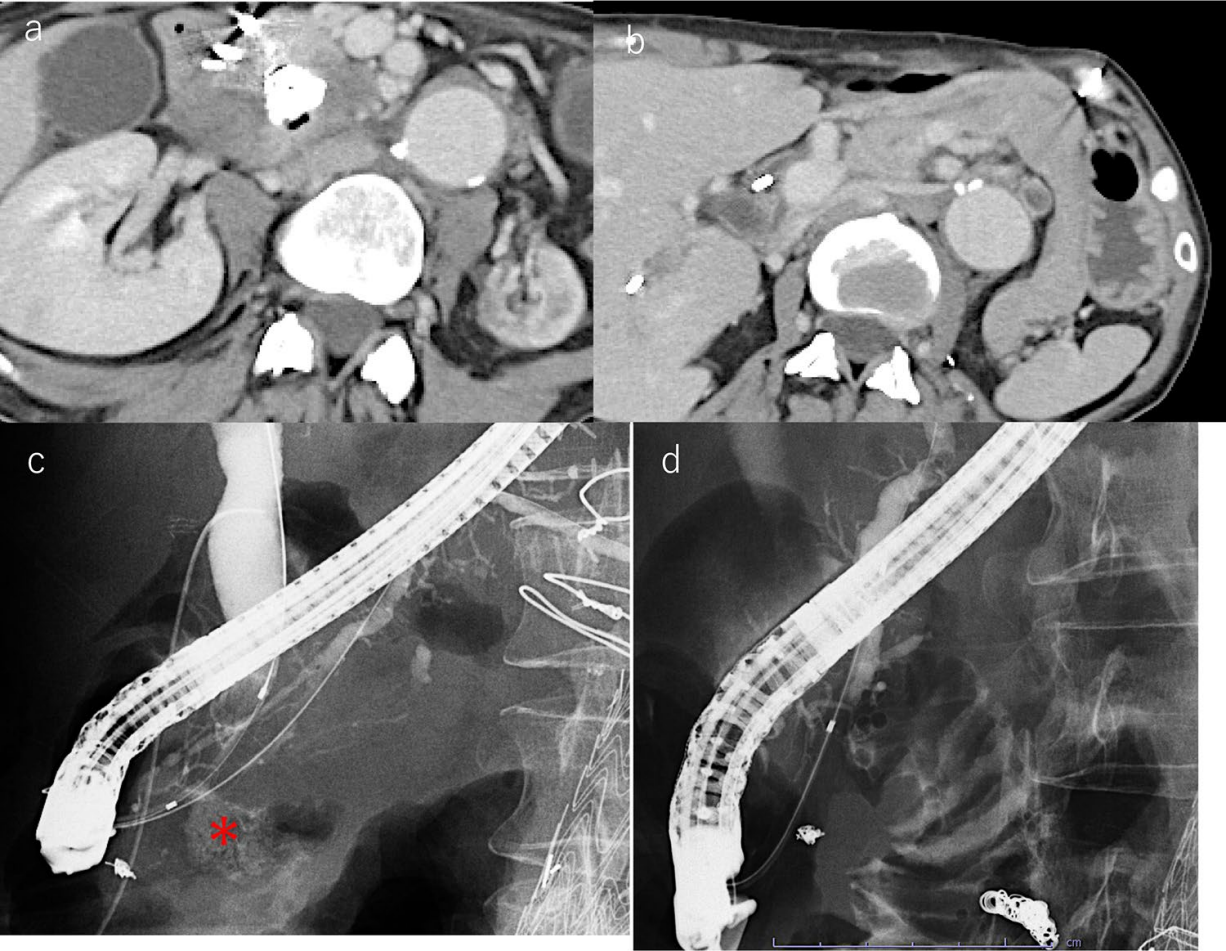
Follow-up images. a Contrast-enhanced CT obtained 4 months after TAE showing marked shrinkage of the pseudoaneurysm. A small amount of air is visible within the lesion, suggestive of fistula formation between the pseudoaneurysm and the duodenum. This finding improved with conservative management. b CT image obtained 4 months after TAE showing improvement in pancreatic swelling, indicating resolution of acute pancreatitis following pancreatic duct stent placement. c Four months after TAE, when contrast material was injected into the pancreatic duct, the post-TAE pseudoaneurysm (*) was opacified through the stenotic main pancreatic duct at the pancreatic head. d One year after TAE, no contrast material leakage was observed
